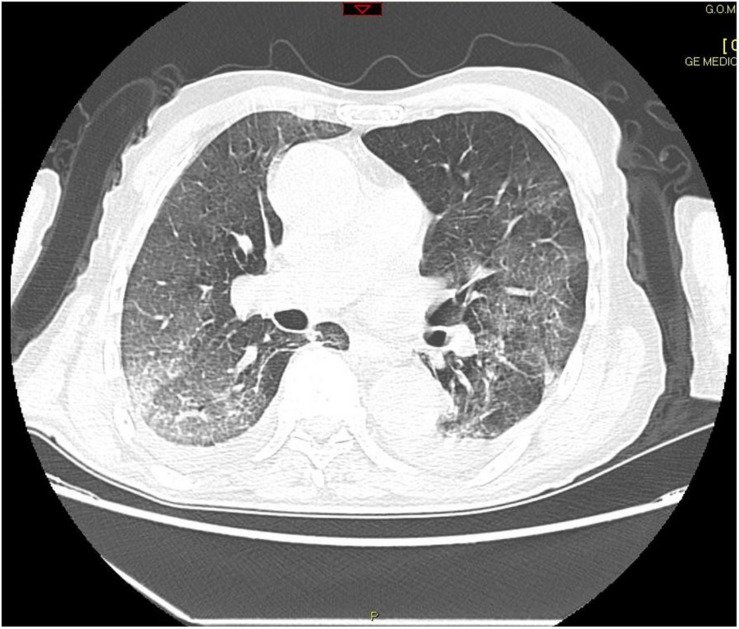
CT scan on day 3 showed bilateral, patchy alveolar opacities progressing to diffuse consolidations, with a “white lung” appearance and widespread ground-glass opacities and moderate bilateral pleural effusions.
